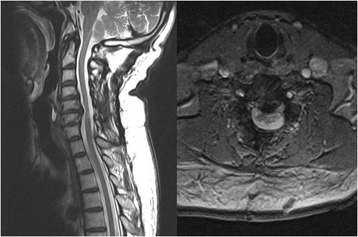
Postoperative magnetic resonance imaging demonstrating extensive decompression
